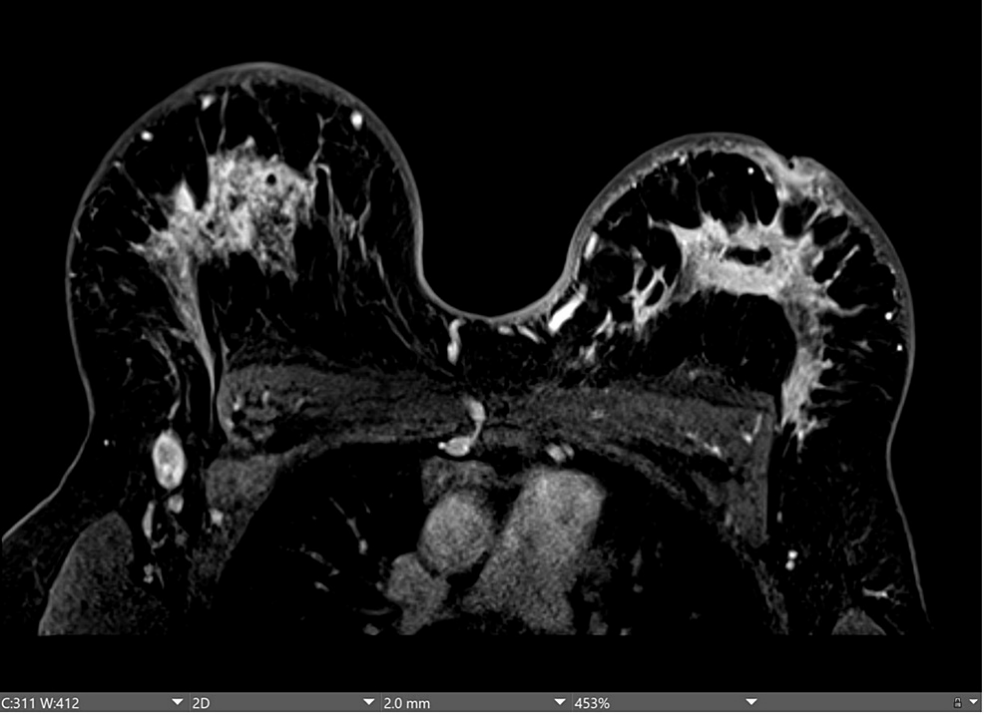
T1-weighted axial contrast-enhanced MRI demonstrating abnormal right axillary lymph node demonstrating rounded morphology. Skin thickening of the right and left breasts demonstrating bilateral breast masses and abnormal enhancement. MRI: magnetic resonance imaging
